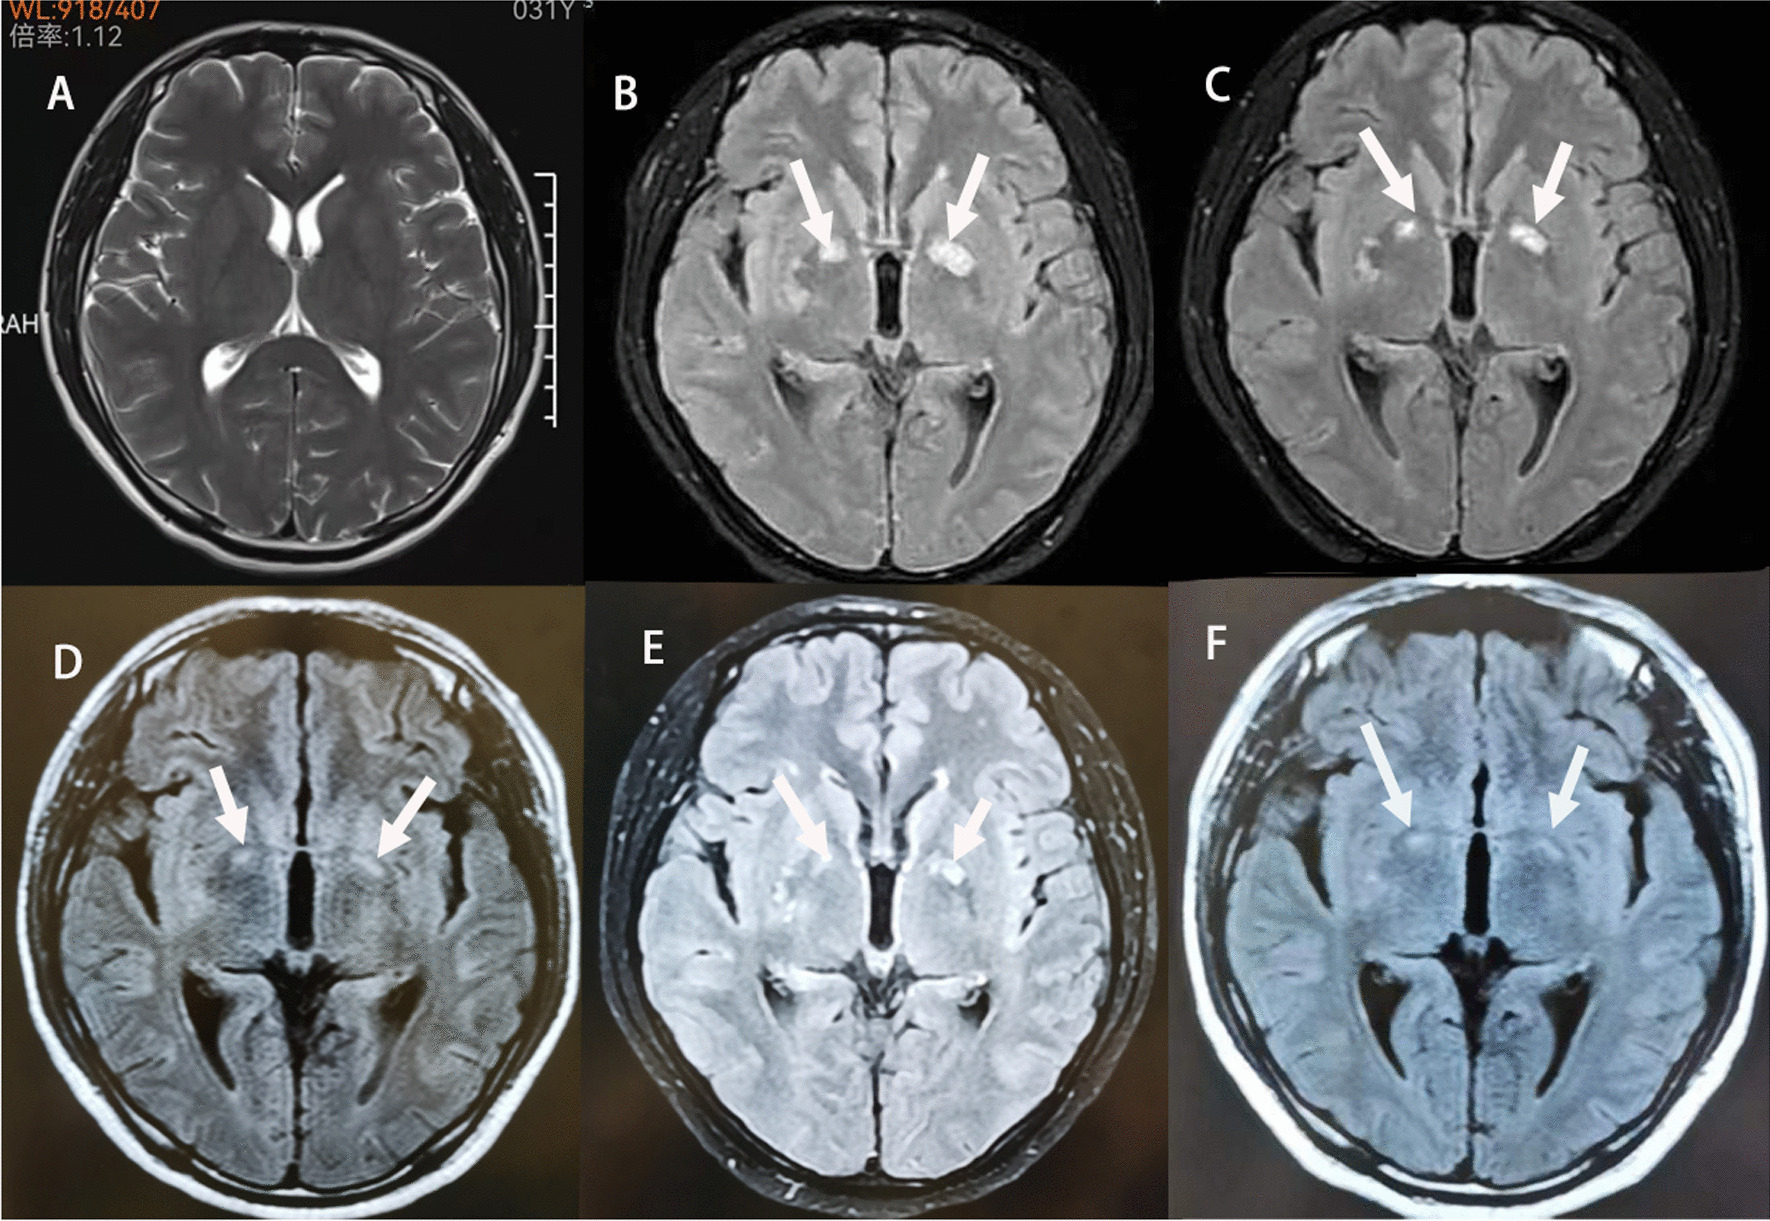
Cranial MRI images A–F. A January 28, 2022—no significant patchy shadows in the bilateral internal capsules and the right basal ganglia. B February 25, 2022—patchy abnormal shadows in bilateral internal capsules and right basal ganglia (arrows), which was diagnosed as Cryptococcal infection. C March 14, 2022—smaller patchy abnormal shadows in bilateral internal capsules and right basal ganglia (arrows). D May 17, 2022—patchy shadows in bilateral internal capsules and the right basal ganglia (arrows). The lesions were smaller than before. E July 6, 2022—patchy shadows in bilateral internal capsules and the right basal ganglia (arrows). The lesion in the right basal ganglia was slightly smaller than before, and the left lesion was roughly similar as before. F September 25, 2022—patchy shadows in bilateral internal capsules and the right basal ganglia (arrows). The lesions were significantly smaller than before. Note: Magnetic resonance imaging
